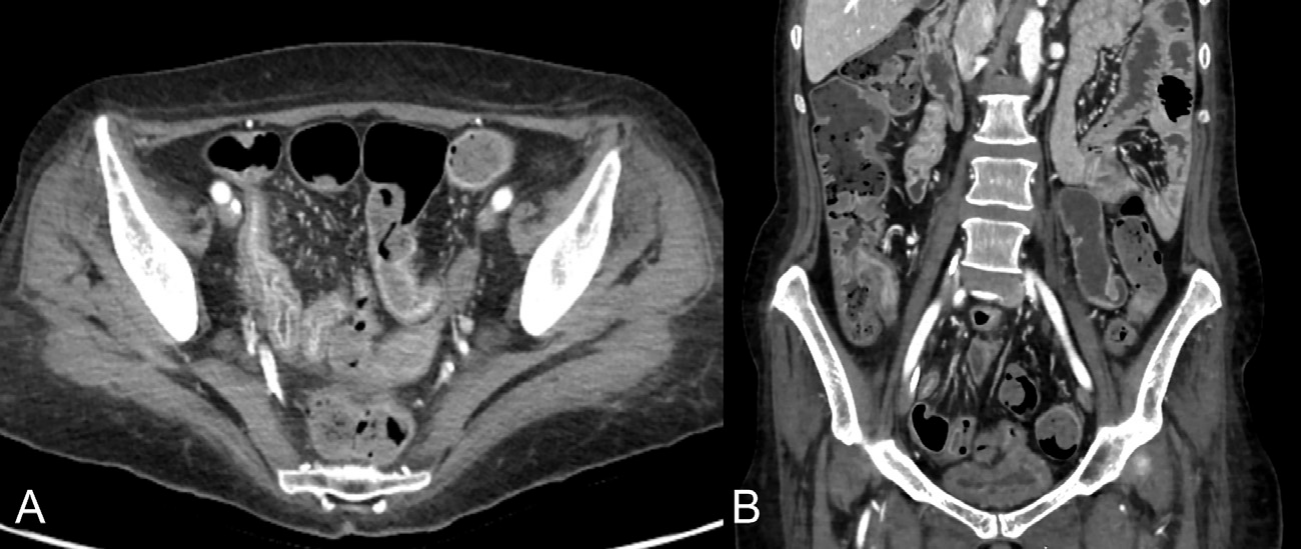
Axial (A) and coronal (B) contrast-enhanced CT images showed bowel wall thickening of the terminal ileum, with mural hyperenhancement and comb sign; these findings are suggestive of inflammatory bowel disease (IBD).
